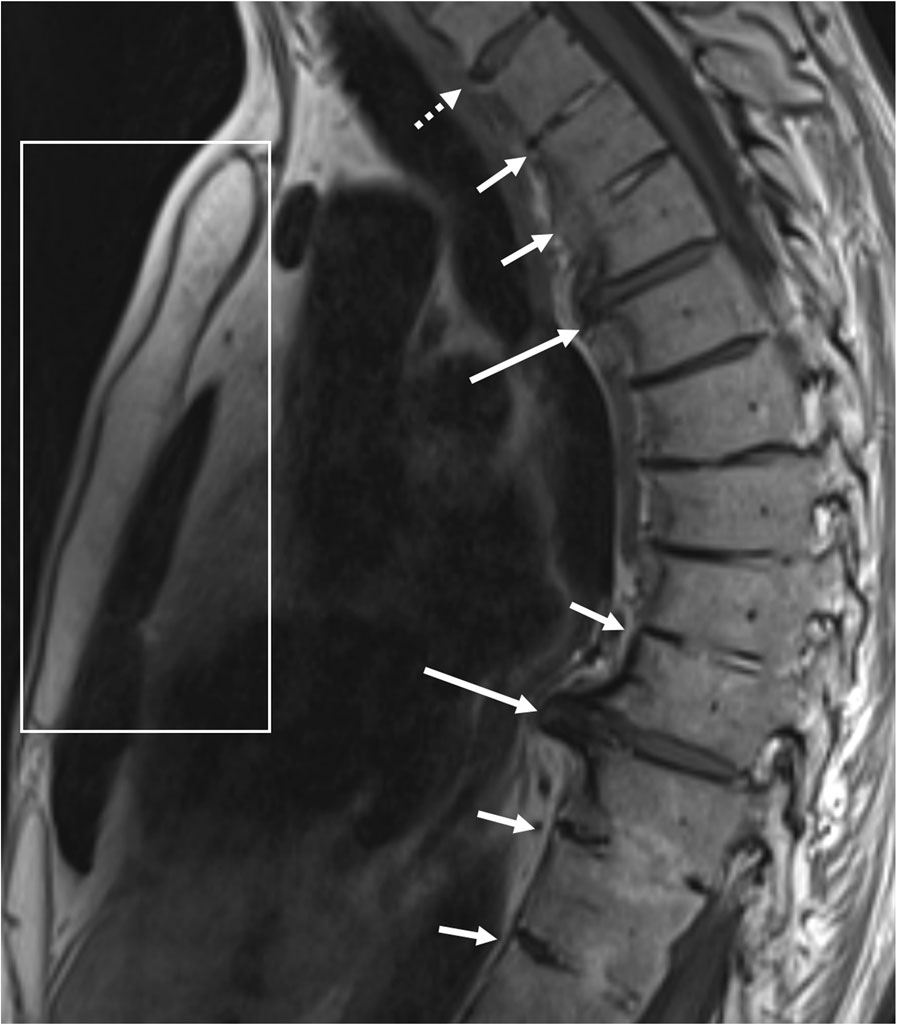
Extensive—and several types of—new bone formation in one patient with SpA. Sagittal T1-weighted MR image shows how bony fusion of the manubriosternal joint can be observed on sagittal imaging of the spine in a routine SpA protocol (rectangle). Also, notice the other types of new bone formation: syndesmophytes (dotted arrow), discal high signal intensity and/ or progressive trandiscal ankylosis (short arrow), and osteophytes (long arrow). This image demonstrates that osteophytes can be seen in patients with SpA at levels otherwise unaffected by SpA, perhaps due to increased mechanical loads on the remaining functional disco-vertebral units
